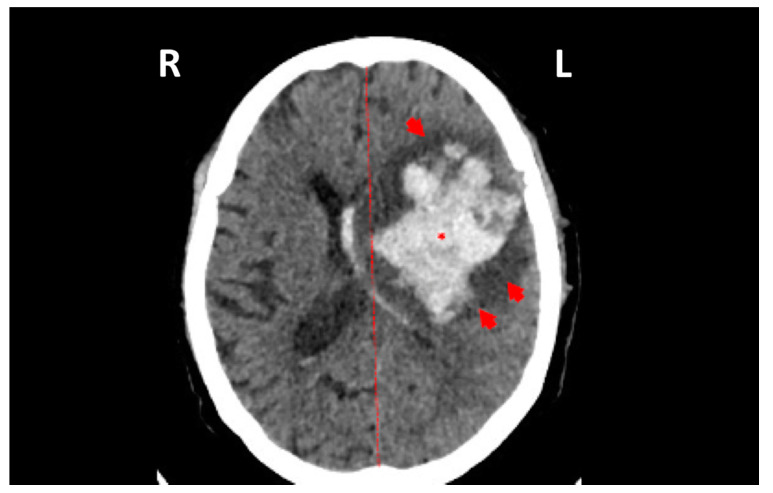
Computed tomography imaging of the patient’s brain showing an intraparenchymal hemorrhage (asterisk) surrounded by a brain edema (red arrows), as well as deviation from the cerebral middle line (red line).
